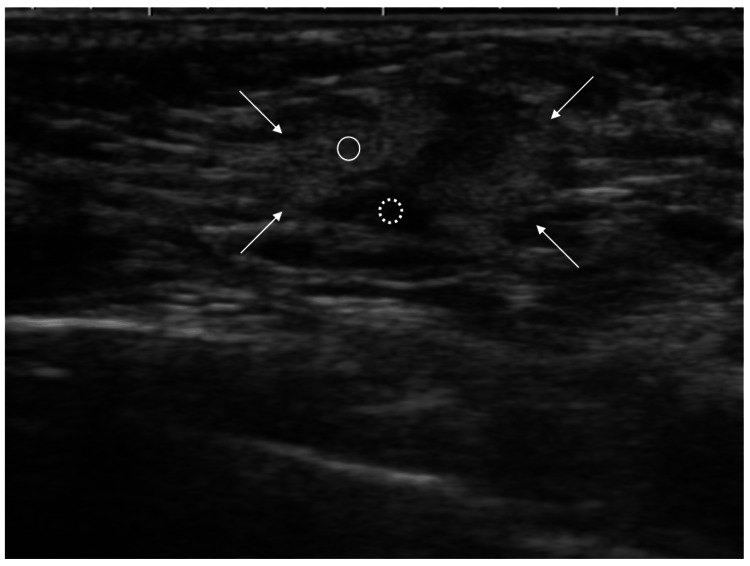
Ultrasound findings Ultrasound showed an oval mass both with indistinct margins (arrows) and mixed high (open circle) and low (dotted open circle) internal echoes in the axilla. When compared with the low-magnified pathological image (Figure 2B), the low echo areas corresponded to fibrous components, while the high echo areas reflected that cancer cells were scattered in fat with low acoustic impedance, generating a large amount of backscattering.
